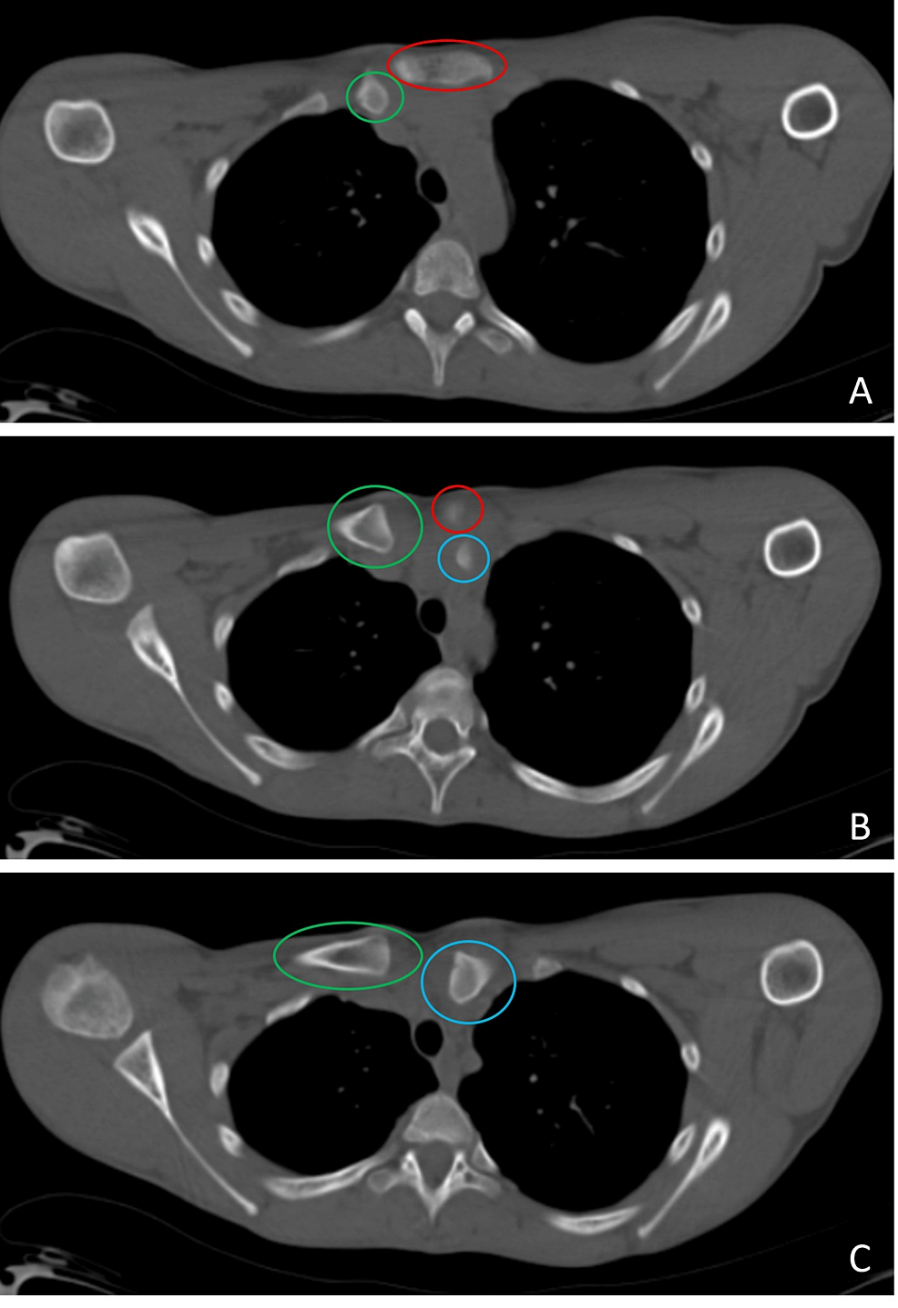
Axial computed tomography sections of the patient after the trauma before the closed reduction The left sternoclavicular joint posterior dislocation is remarkable with the consecutive axial computed tomography sections (A, B, C) after the trauma before the closed reduction. The proximal end of the left clavicle is marked with a blue circle. The proximal end of the right clavicle is marked with a green circle. The sternum is marked with a red ellipse and circle.
